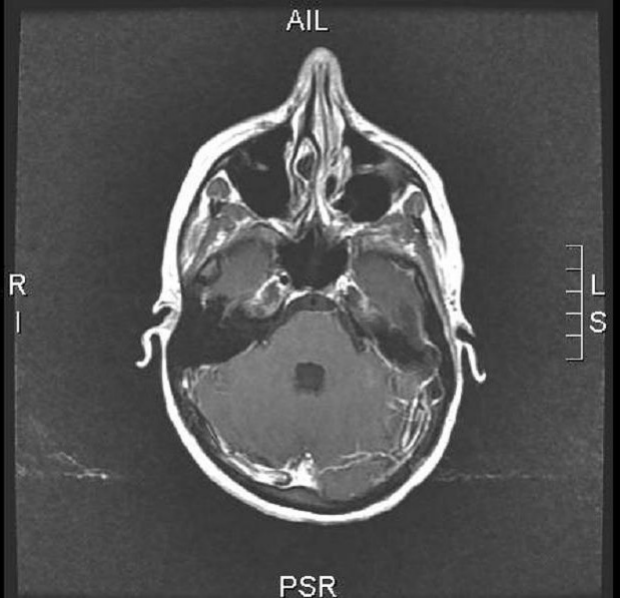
Cerebral angio-MRI after injection of 20 cc of gadolinium in a woman aged 24 with EDS type IV displaying a dissecting haematoma of the left internal carotid.
